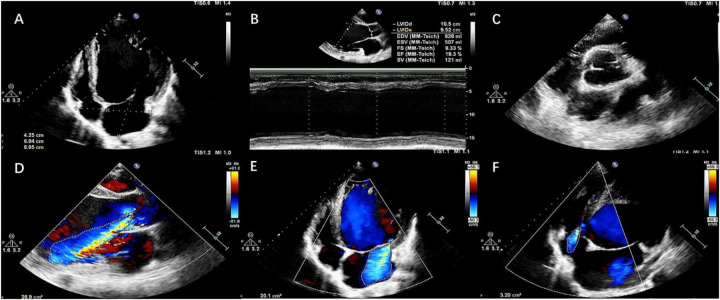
Preoperative transthoracic echocardiography findings. (A,B) Dilated left ventricle (LVEDd 105 mm), left atrium and reduced LVEF (19.3%). (C) The left and right coronary cusps of the aortic valve are fused, leading to functional bicuspid morphology. (D–F) severe eccentric aortic regurgitation, severe mitral regurgitation and mild tricuspid regurgitation.
